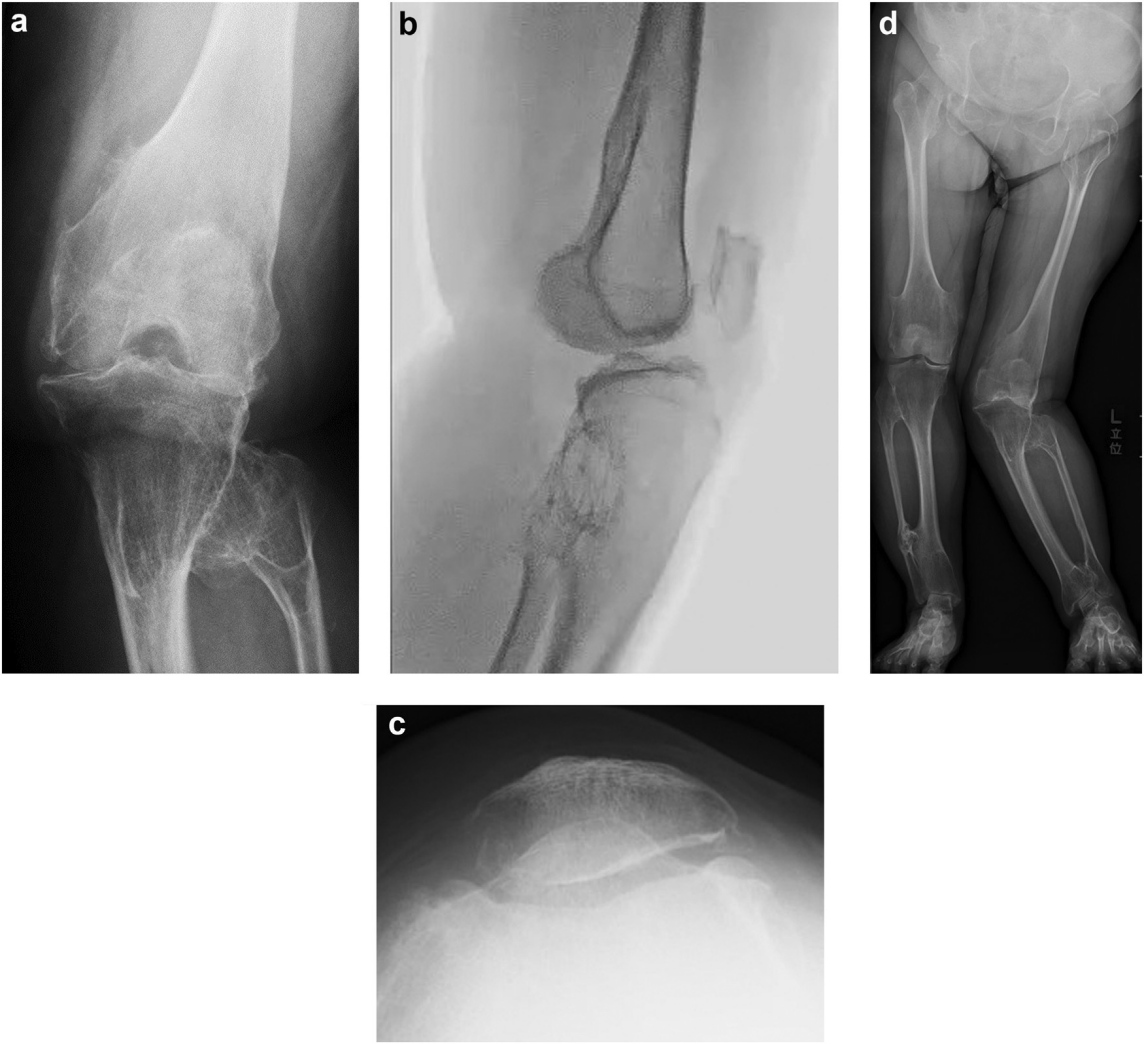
Preoperative (a) anteroposterior, (b) lateral, and (c) skyline views and (d) full-length standing radiographs. (a) The left knee contained exostoses, and end-stage osteoarthritis was present. (b) Femoral posterior subluxation caused by the posterior tibial slope was evident in the lateral view. (c) Patellar dislocation was not present in the skyline view. (d) Full-length views of the lower extremities demonstrated 43-degree valgus deformity of the left knee.
